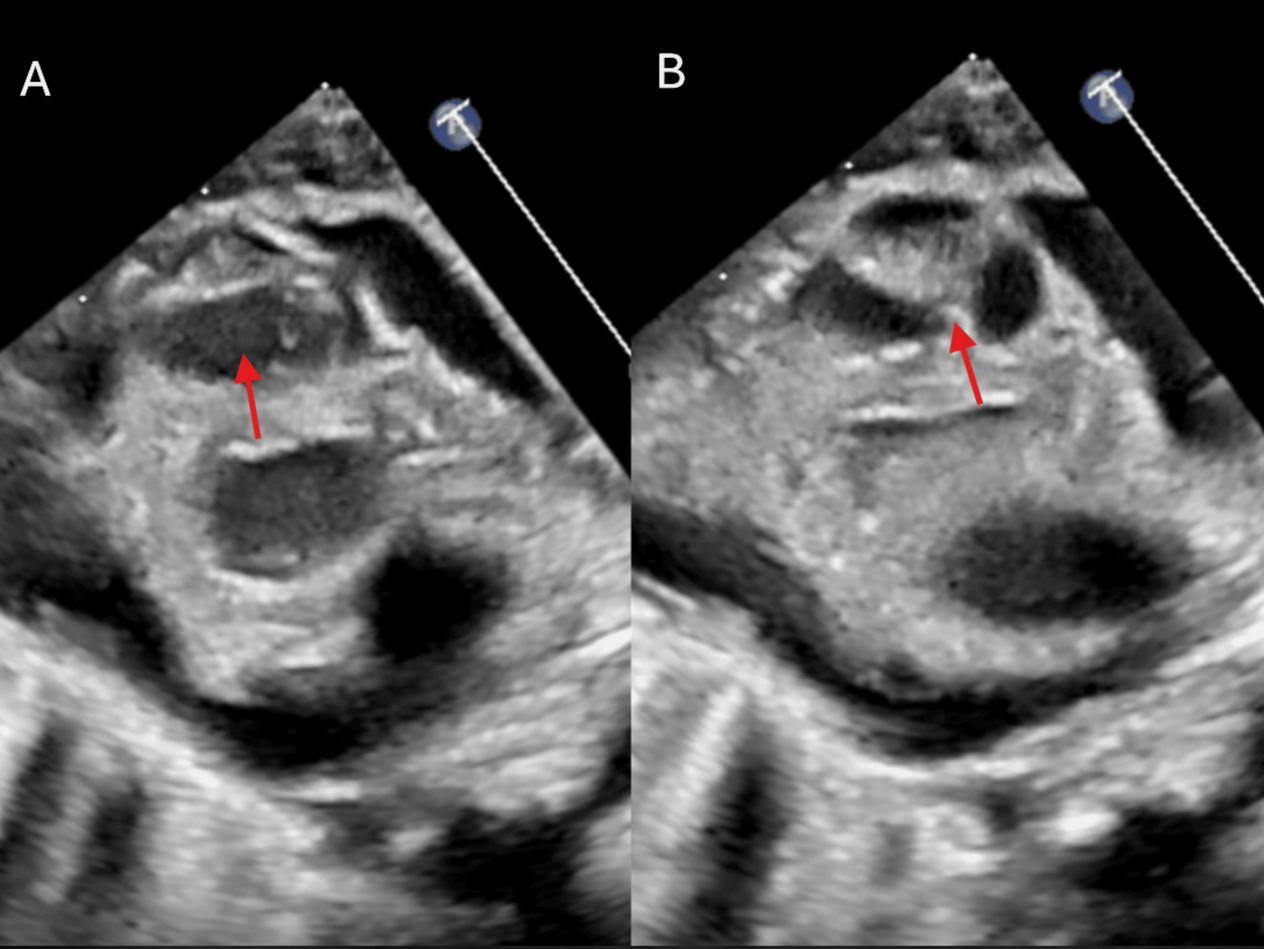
Parasternal long-axis view demonstrating thickening of the aortic valve leaflets, evident during both (A) systole and (B) diastole (arrow).
